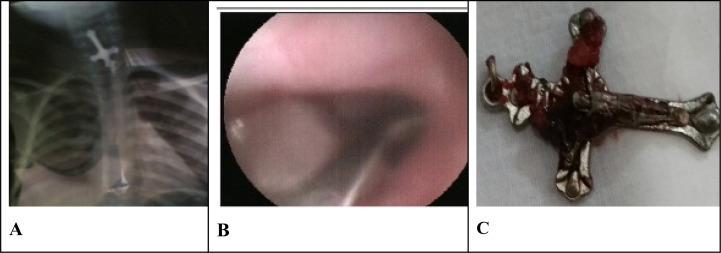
(A) chest X-ray, (B) endoscopic image (C)same crucifix after endoscopic removal from a 6-year-old girl
